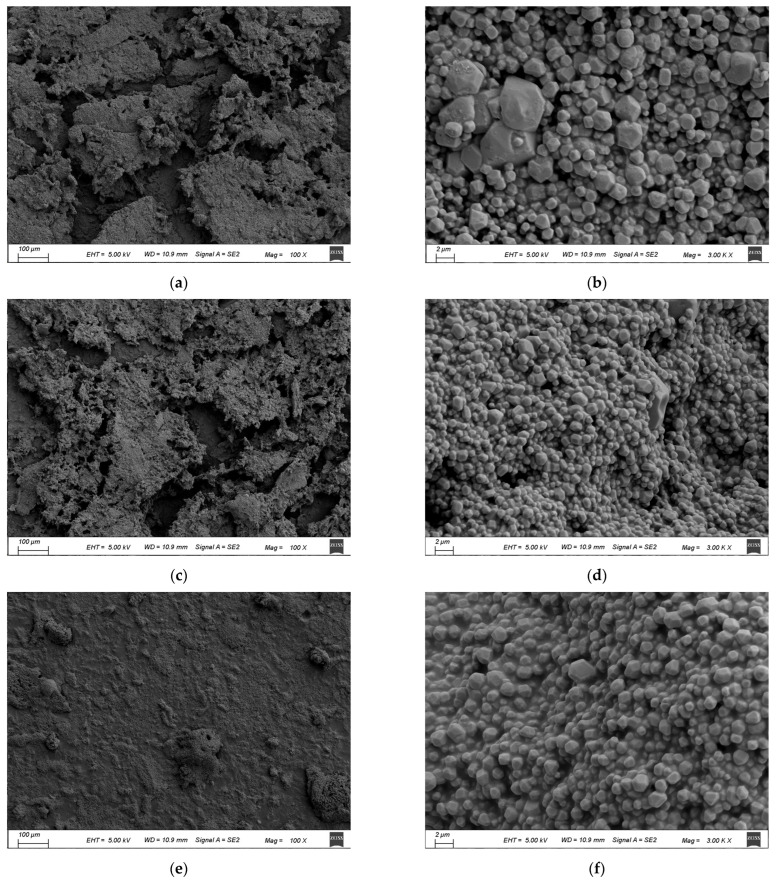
SEM images of CCHS prepared in different ultrasound-assisted modes: (a,c,e) represent CE, UPH, and WUH with 100× magnification; (b,d,f) represent CE, UPH, and WUH with 3000× magnification.
